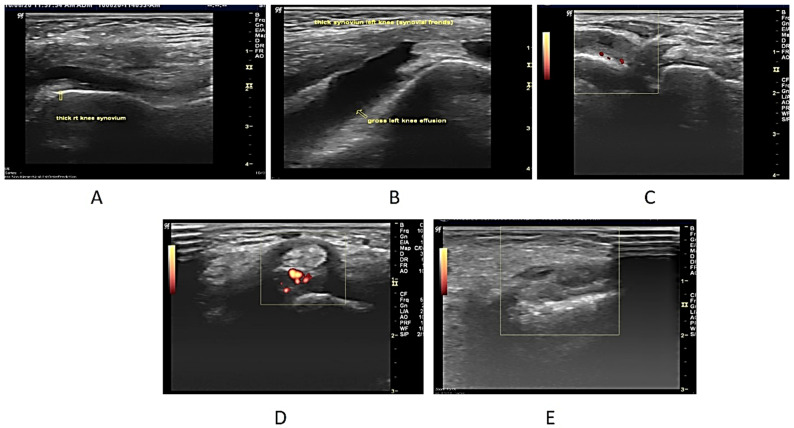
(A). Longitudinal USG image of the right knee in a 7-year-old male patient who presented with bilateral knee swelling and mild fever. ESR was also raised. On ultrasonography, both knees revealed synovial hypertrophy with mild effusion in the right knee joint (arrow). (B) Longitudinal USG in a 12-year-old girl child presenting clinically as a case of multiple joint pain and swelling in both knees. On examination, both knees were swollen. ESR and CRP were raised. The ultrasonographic image reveals Joint effusion with Synovial hyperplasia confirming clinical findings. (C) USG image in a 6-year-old Male child with clinically diagnosed JIA having active findings of joint tenderness and elevated local temperature at 2nd MCP. The image reveals synovial hyperplasia with increased vascularity in the thickened synovium on the Power Doppler (Pulse repetition frequency (PRF), which indicated the number of ultrasound pulses emitted by the transducer over a designated period of time of 0.7 kHz. (D) Transverse USG image of the posterior tibial tendon in a 10-year-old male patient of JIA showing peri-tendon synovial thickening and increased vascularity suggestive of tenosynovitis. (E) USG image at the level of the wrist joint in a 10-year-old male child diagnosed with JIA with no evidence of wrist joint involvement clinically showing evidence of synovial hyperplasia (arrow). Power Doppler showed a lack of flow.
